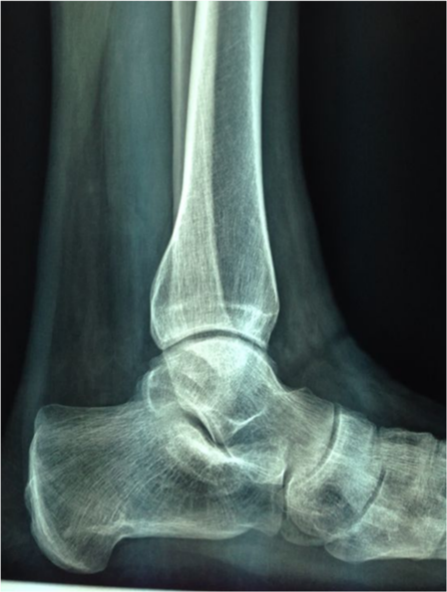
Lateral radiograph of the left ankle of the same patient at 7-year follow-up, showing no progression of degenerative changes
